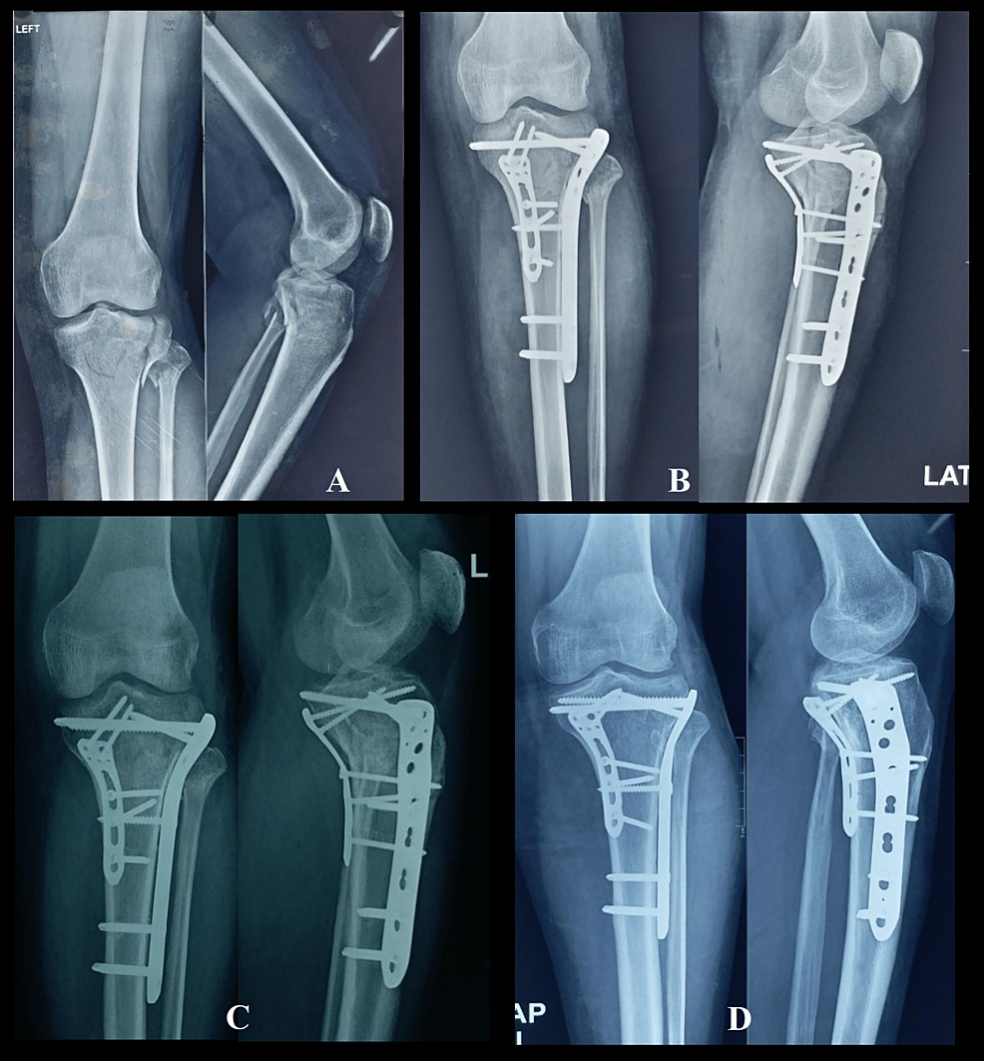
Serial follow-up radiographs of a 28-year-old male with complex proximal tibial plateau fracture of the left side managed by dual plating using two separate incisions. A: Pre-operative radiographs showing the sustained fracture pattern.
